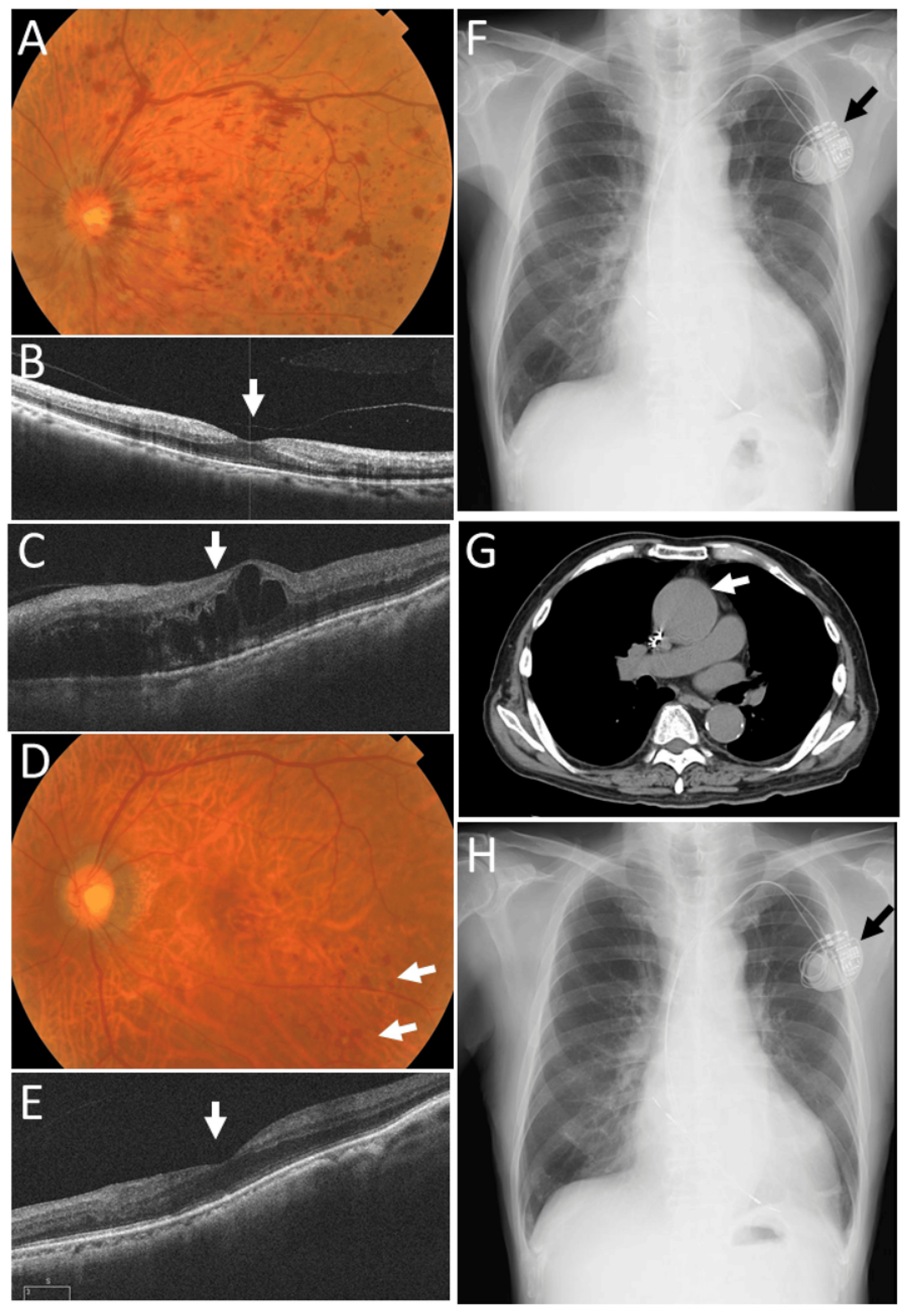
Ophthalmic images, plain chest X-rays, and chest CT at age 72 years Fundus photograph and horizontal OCT image of the left eye at the onset of left CRVO at age 72 years, showing a limited number of flame-shaped and blot retinal hemorrhages with venous dilatation (A) and no macular edema (arrow, B). Horizontal OCT image obtained two months later, immediately after panretinal laser photocoagulation, demonstrating cystoid macular edema (arrow, C). Fundus photograph and horizontal OCT image obtained 10 months later, five months after cardiac surgery, showing a small number of residual blot retinal hemorrhages (arrows, D) and resolution of macular edema (E). Plain chest X-ray films obtained two weeks before the onset of left CRVO (F) and two months later (H) show no change in cardiomegaly, which was greater than that observed one year earlier (Figure 1C, 1D). Chest CT obtained one month later shows no change in the fusiform ascending aortic aneurysm (arrow, G). Black arrows in F and H indicate the heart pacemaker. CRVO, central retinal vein occlusion; OCT, optical coherence tomography
